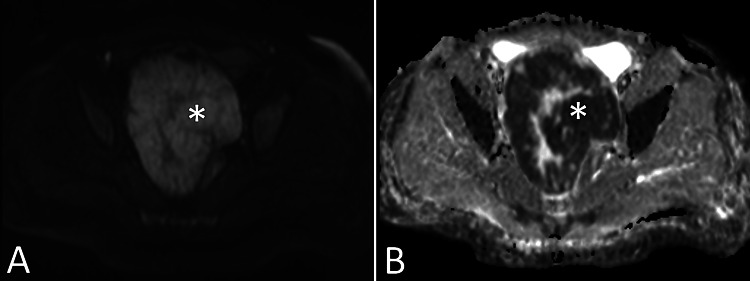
Diffusion weighted pelvic MRI. Axial diffusion weighted imaging (A) and ADC map (B) from the pelvic MRI demonstrate diffusion restriction of the solid portions of the pelvic mass (asterisks), with hyperintensity on diffusion weighted imaging and corresponding hypointensity on ADC. ADC: Apparent diffusion coefficient
